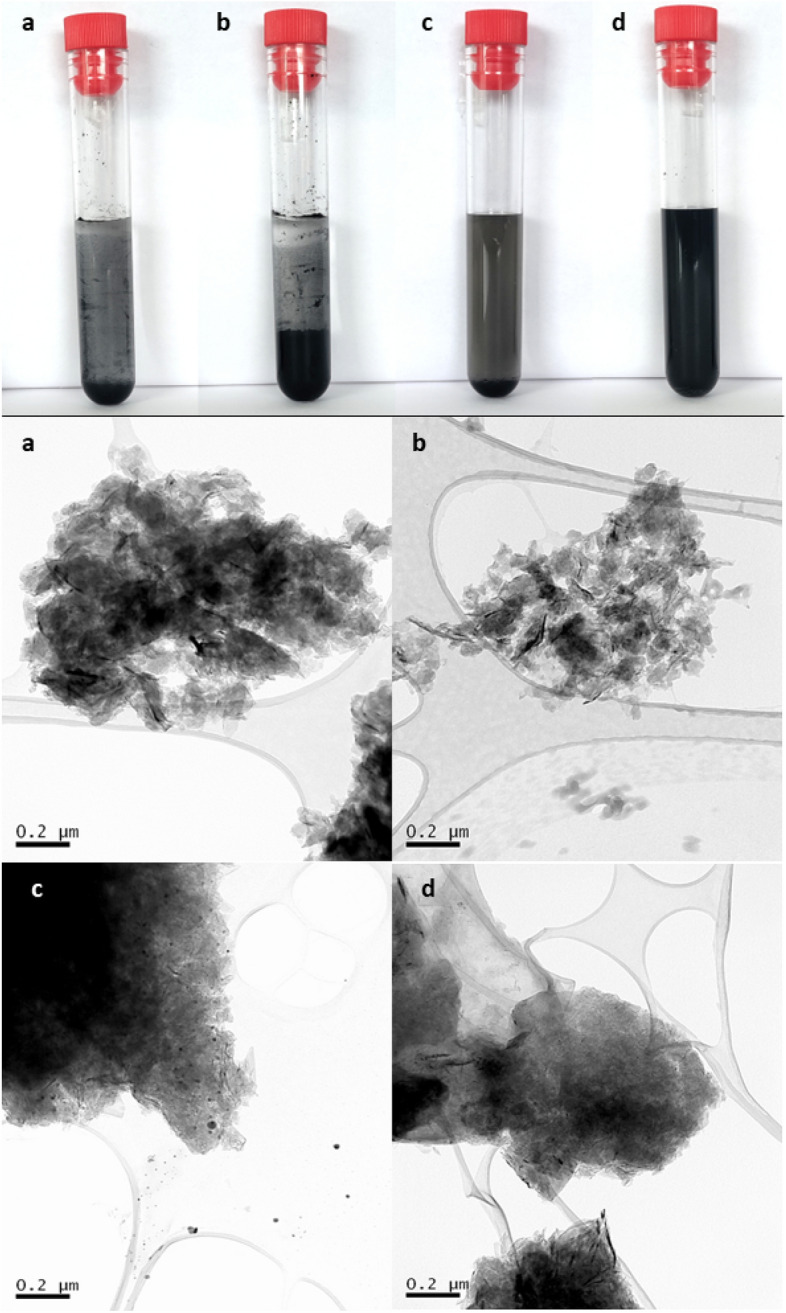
Images of the NMs used in this study. Upper panel: pictures of the NMs in suspension. Lower four panels: TEM analysis of pristine and ultrasonicated materials. Key: Pristine G2 (a), ultrasonicated G2 (b), pristine GNA15 (c), ultrasonicated GNA15 (d).
